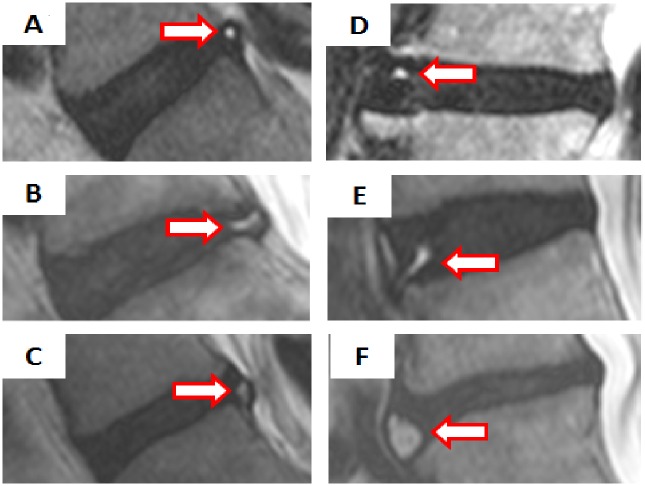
Classification of High Intensity Zones based on morphology and topography. High Intensity Zones (HIZ) were defined as a high intensity signal (white) surrounded by low intensity (black) located in the annulus fibrosus on T2-weighted sagittal MRI. Six types of HIZs were created based on the shape (round type, fissure type, vertical type, rim type, and giant type), and location within the disc (posterior or anterior). The images represent (A) posterior round type, (B) posterior fissure type, (C) posterior vertical type, (D) anterior round type, (E) anterior rim type, and (F) anterior enlarged type.
